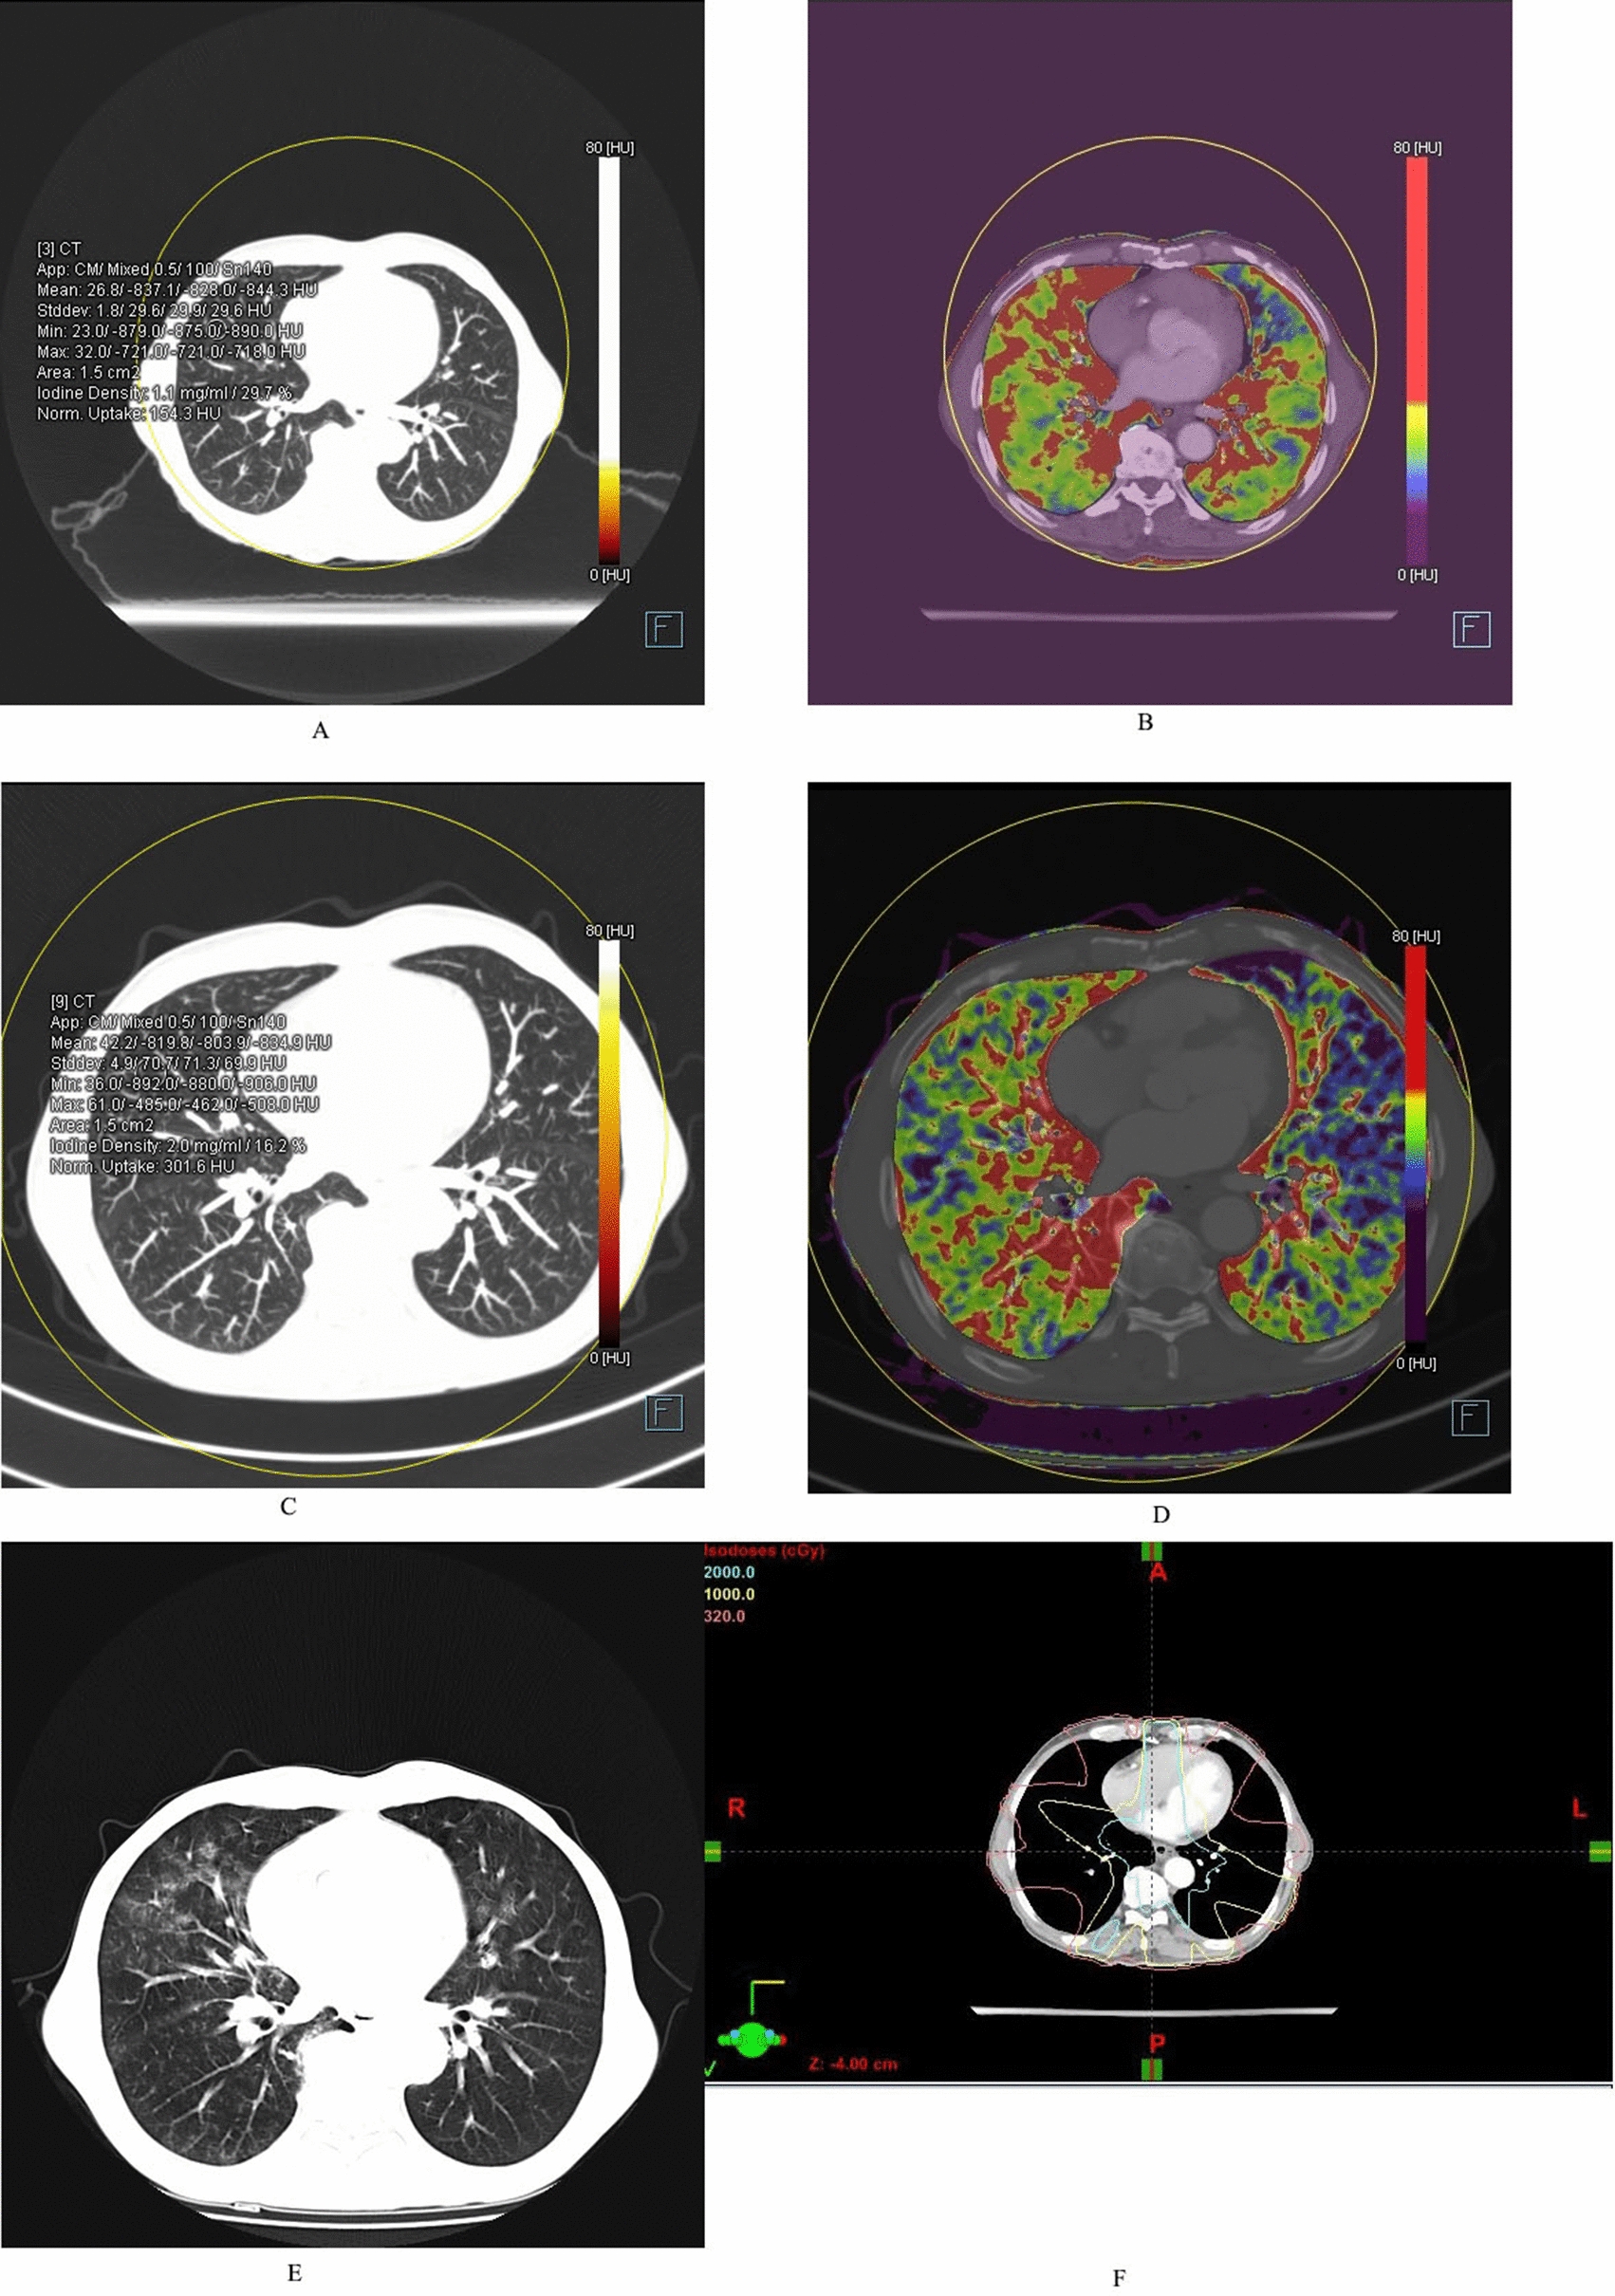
Male/64 years old, middle segment esophageal cancer; a Dual-energy lung window image before radiotherapy, the NIC value of the right middle lung is 0.297; b Lung PBV color image before radiotherapy, blood flow (red part) is more abundant in bilateral irradiated fields; c Dual-energy lung window image after radiotherapy, the NIC value of the right middle lung is 0.162; d Lung PBV color image after radiotherapy, blood flow (red part) is less than before; e CT image during follow-up period, ARP is seen in both lungs; f TPS transverse localization image, the irradiation range was within the red line, and ARP is seen in the irradiation areas of V_> 20 Gy_, V_10-20 Gy_, and V_5-10 Gy_
